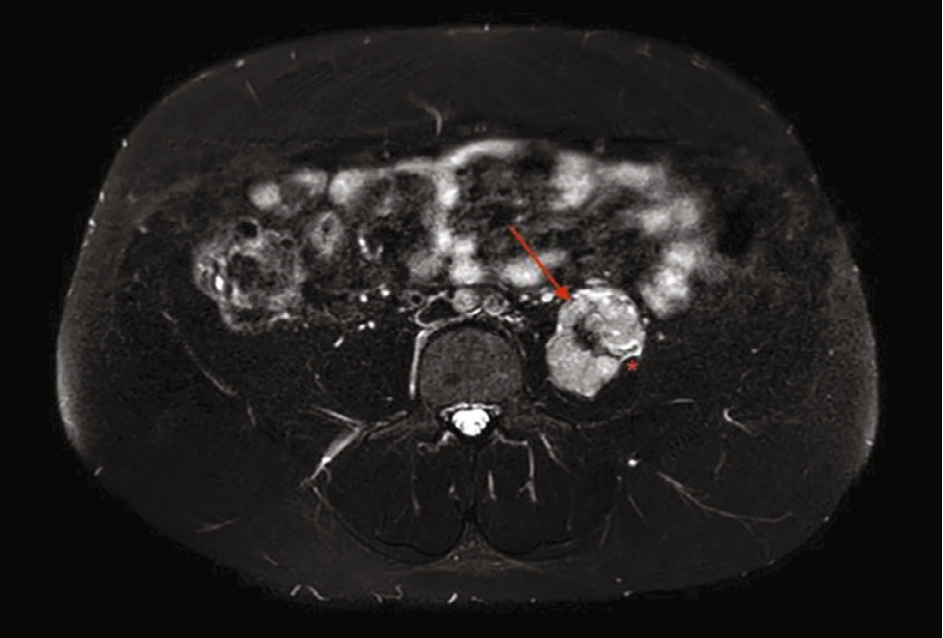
The red arrow showing lobulations and calcifications within the mass, asterisk showing the junction of tumor and psoas muscle (axial T2-weighted MRI).
